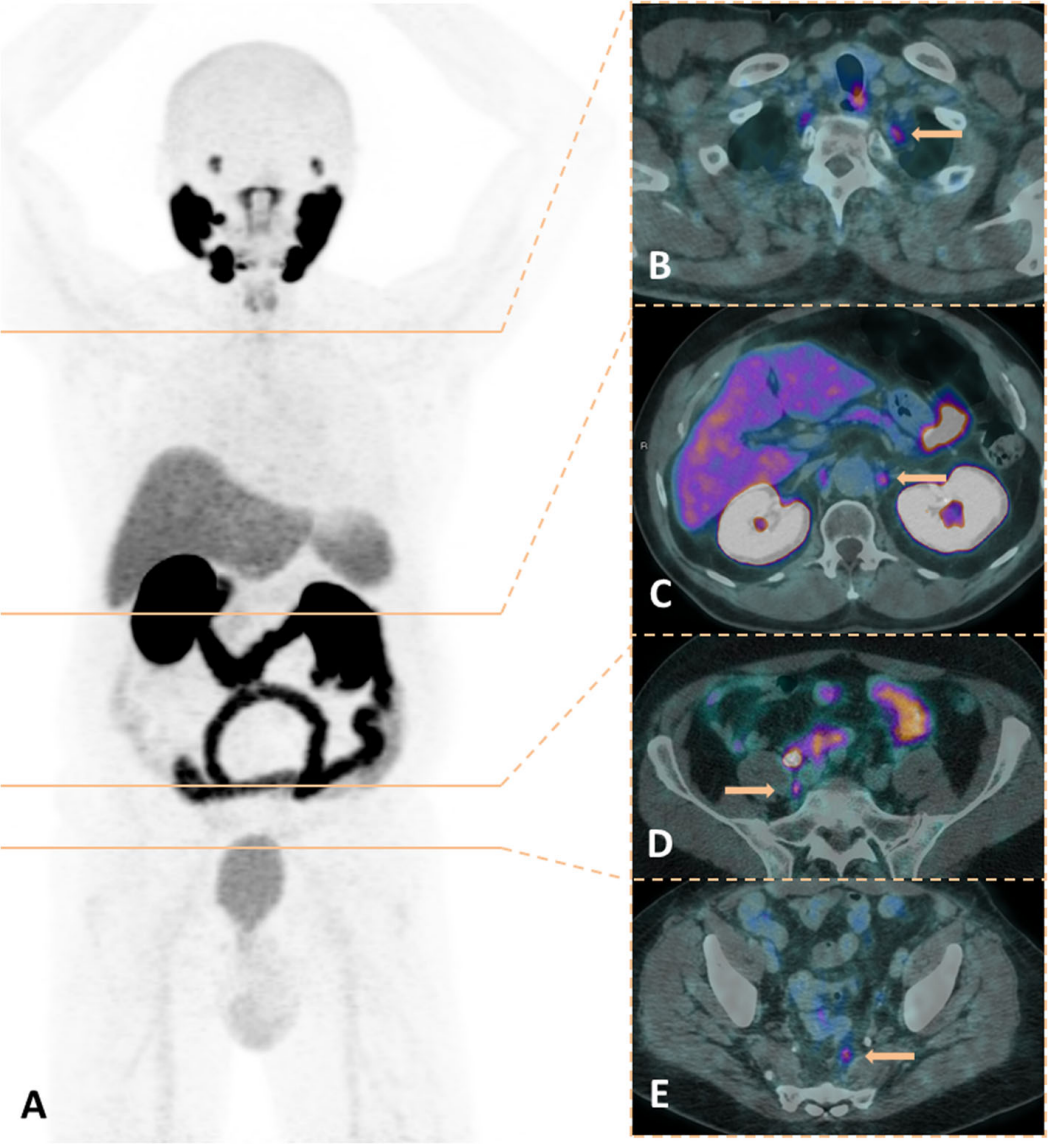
An overview of physiological PSMA uptake in sympathetic ganglia. ^68^Ga-PSMA-PET MIP (a) with corresponding levels on fused PET/CT images (b, stelate ganglia; c, celiac ganglia; d, hypogastric ganglia; e, sacral ganglia)
